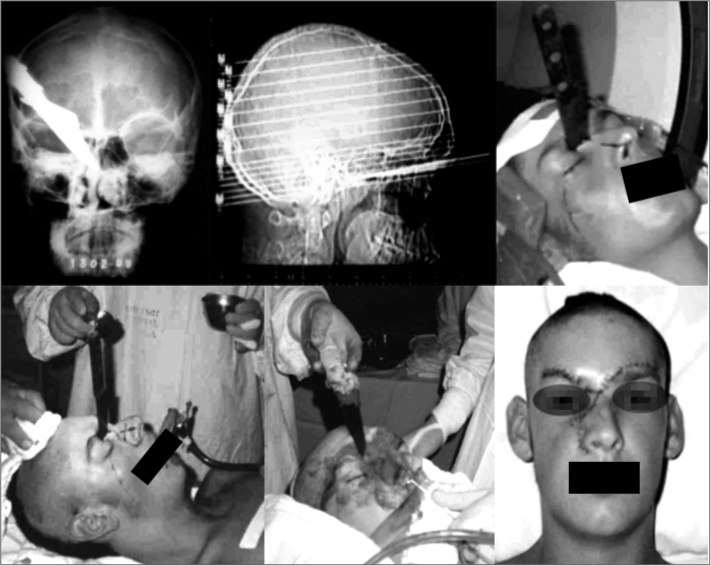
Presentation of the anatomical positions of the foreign body in radiographs and tomography, aspect of the skin, the surgical access, and the patient in the immediate postoperative period.
